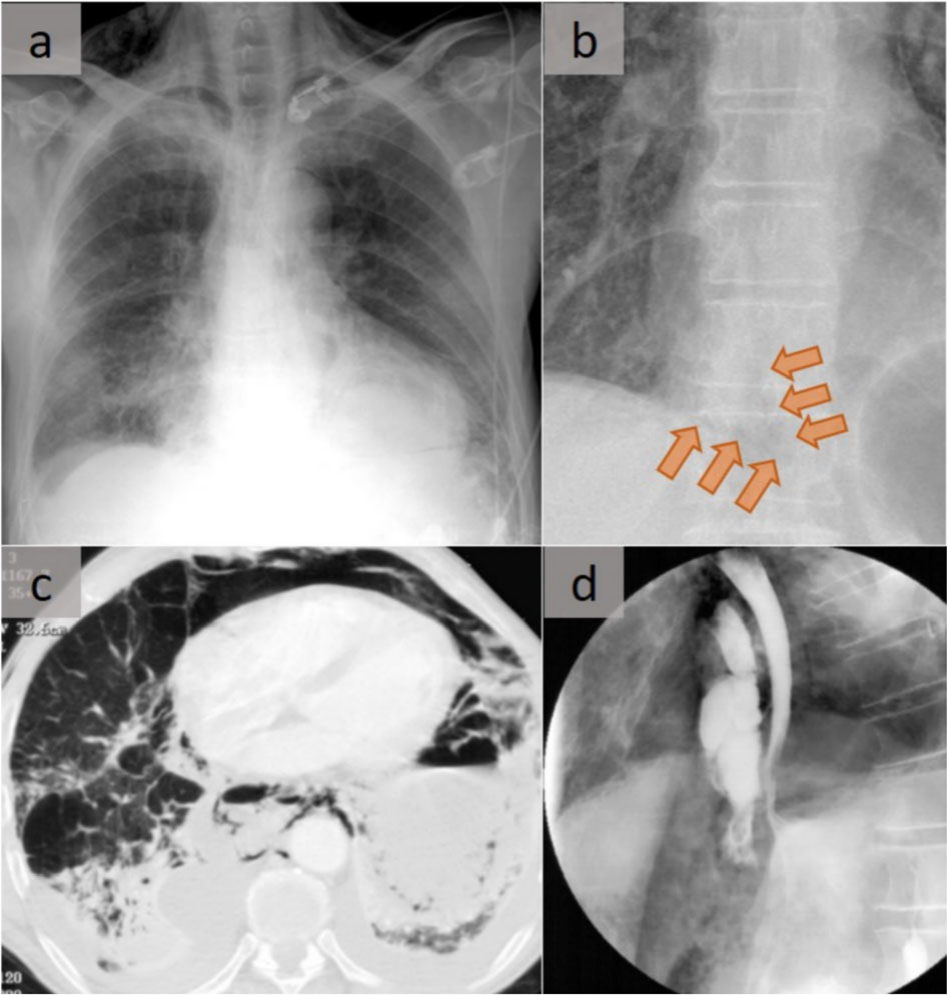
Boerhaave’s syndrome related to esophageal rupture. a Chest X-ray shows pneumomediastinum with subcutaneous emphysema. b Naclerio V sign in another patient. In this case, the Naclerio V sign was the only finding that raised the suspicion of an esophageal rupture (not shown) with a focal and limited pneumomediastinum. c Axial CT scan in lung windowing that shows the pneumomediastinum with bilateral pleural effusion and alveolar consolidation (d). Iodine hydro-soluble contrast on a lateral view shows extravasation above the diaphragm in front of the esophagus (Patient (a))
